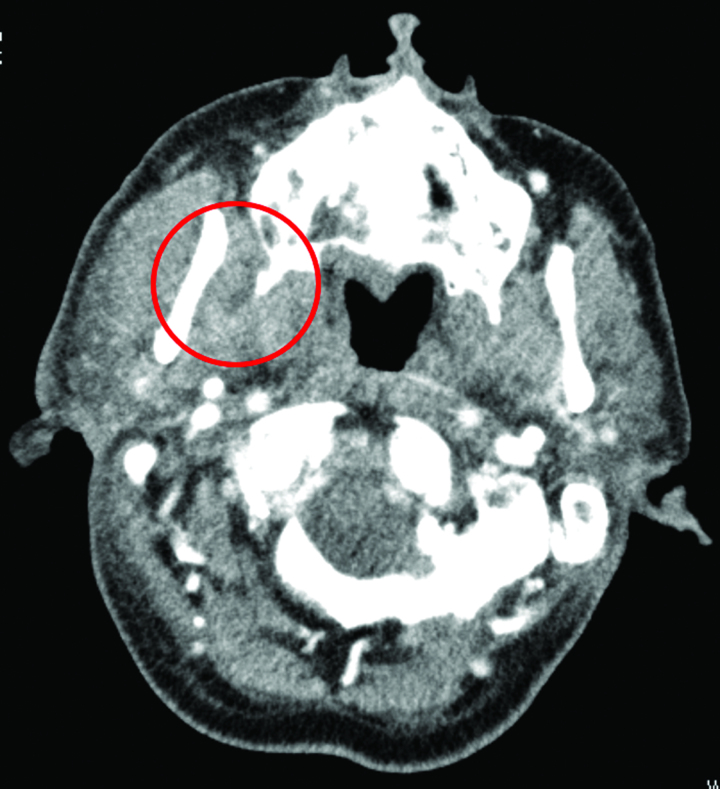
Contrast-enhanced CT findings on the first day of admission. An abscess formed between the mandibular branch and the pterygoid process. Buccal cortical bone resorption was observed at the root apex of the maxillary second molar, adjacent to the abscess. CT = computed tomography.
